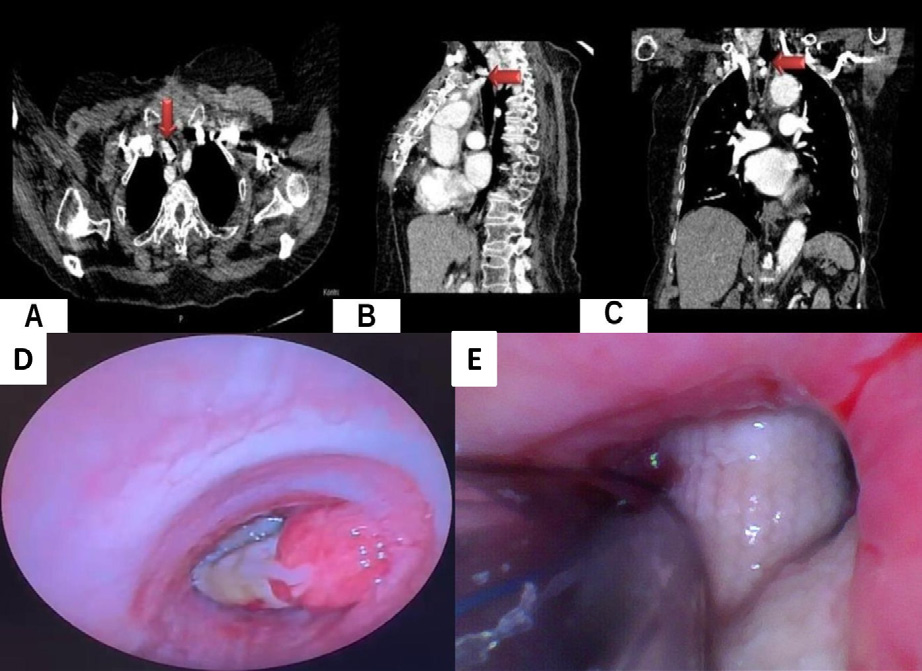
A-C) Contrast-enhanced computed tomography of the chest shows an aorta-carotid bypass graft perforating the right anterolateral wall of the trachea, occluding the tracheal lumen, D) Preoperative video-bronchoscopy showing intratracheal aorta-carotid bypass graft significantly obstructing the tracheal lumen, E) Standard tracheal tube (6.0 mm ID) passing over the prosthetic vascular graft in the trachea.
